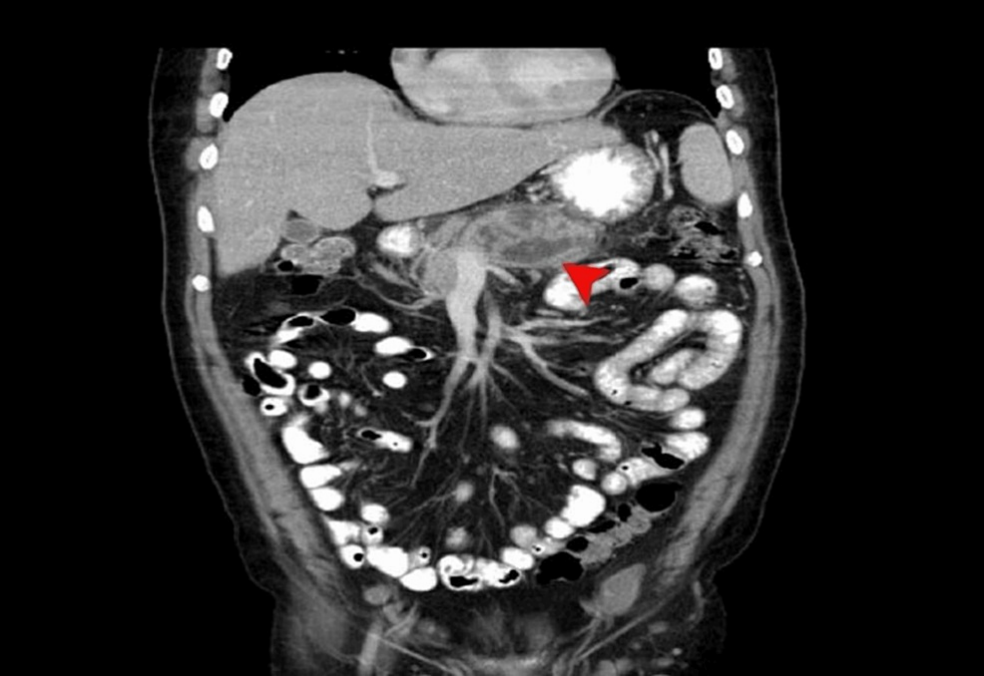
CT abdomen and pelvis with contrast (coronal view) The red arrow represents a mixed density heterogeneously enhancing mass with large areas of necrosis in the body of the pancreas with encasement of the splenic artery and vein likely representing neoplastic pancreatic body mass - mucinous cystadenocarcinoma.
